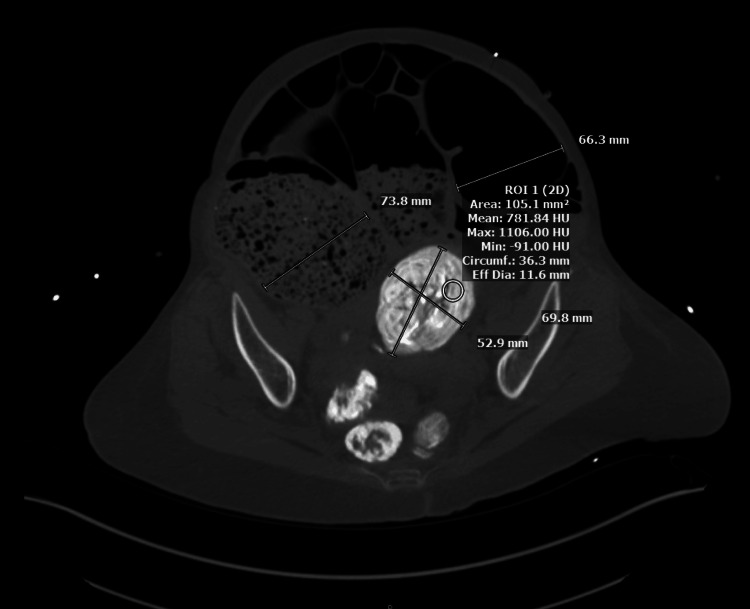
CT imaging in the axial plane revealing a large barolith lodged in the sigmoid colon with upstream dilation of the cecum and transverse colon, and the distal sigmoid is collapsed
